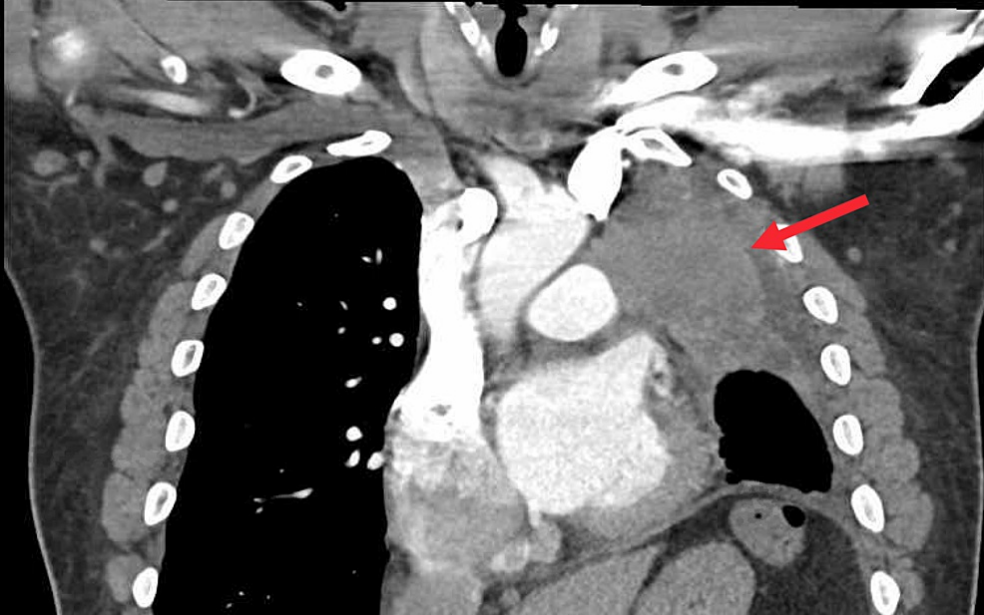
CT scan of the chest demonstrating lung mass compressing on the patient’s left atrium. The arrow indicates the mass.
